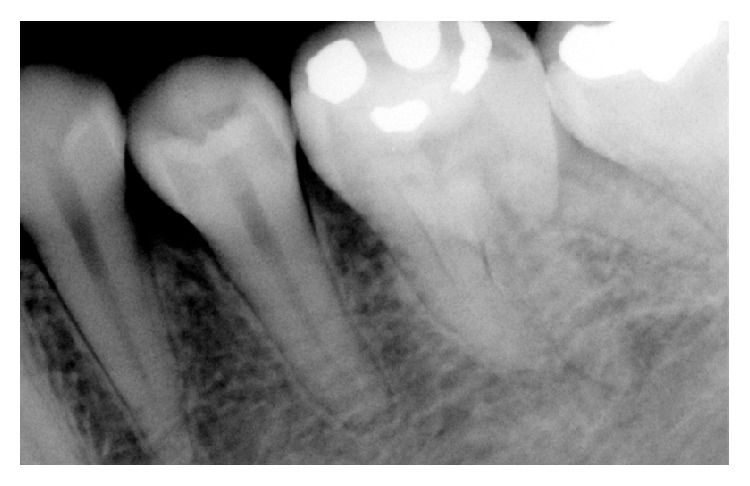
Preoperative radiograph showing three separate roots of mandibular first molar.
